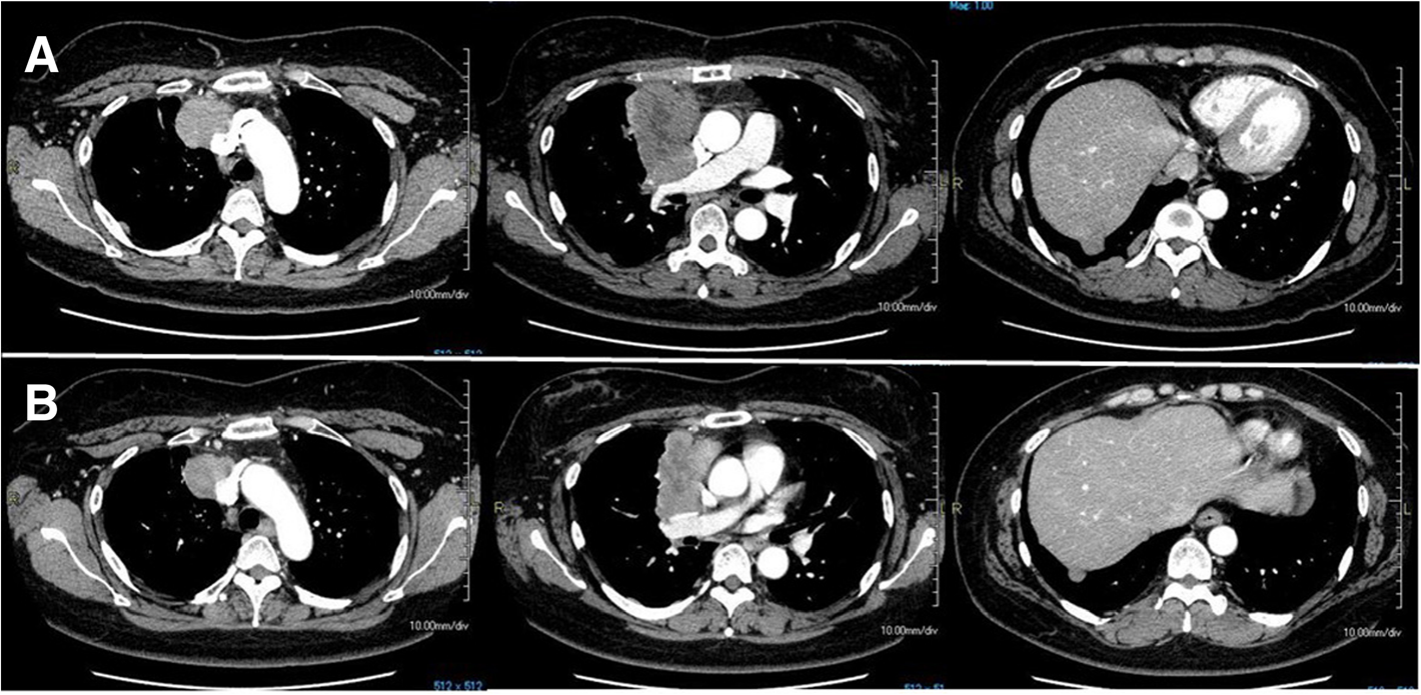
a Chest CT scan showing an anterior mediastinal tumor that is tightly adherent to the superior vena cava (SVC) and pulmonary trunk with swelling of the tracheobronchial lymph nodes and multiple pleural masses. b Chest CT scan showing a regression of the mediastinal and pleural masses after induction chemotherapy
